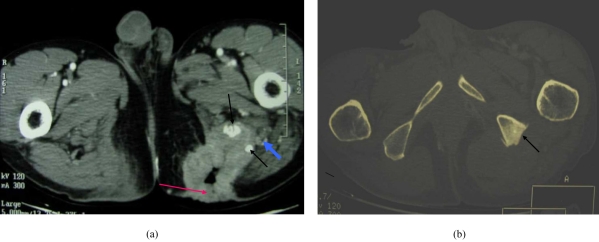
(a) Contrast-enhanced axial CT scan of the pelvis demonstrates an irregular nodular enhancing rim of the ulcer in the left gluteal region, with air pockets and hyperdense foci suggesting bone fragments (short arrow) within it. The sciatic nerve is displaced posteriorly and separate from the tumour (blue arrow); (b) Contrast-enhanced axial CT scan of the pelvis at a higher level (displayed in bone window), showing erosion and sclerosis of the left ischial tuberosity (arrow). There is an associated periosteal new bone formation.
